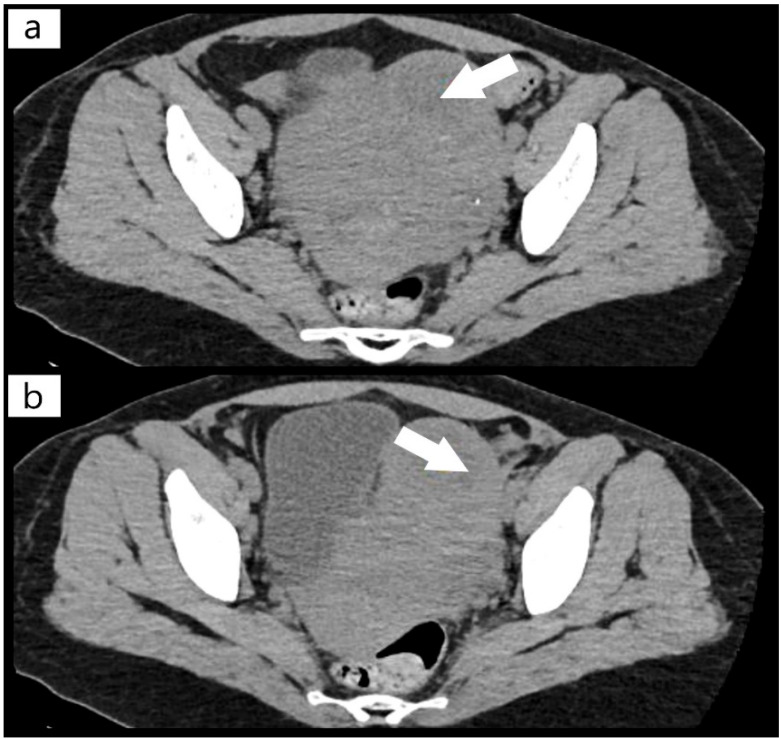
(a,b) Computer tomography (CT) scan with fibroid mass of the uterus (arrows).
